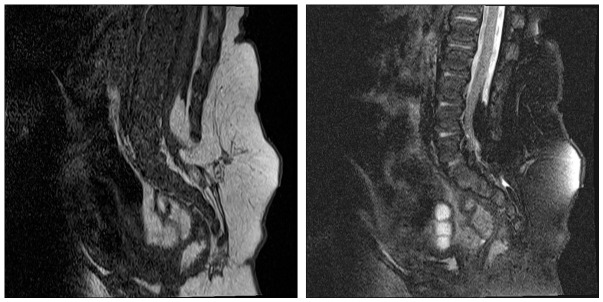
Pre-operative sagittal magnetic resonance imaging of the lumbosacral spine. Left: T1-weighted image, demonstrating the high-signal subcutaneous lipoma extending posteriorly from the spinal canal through a fascial defect; the lipoma is in continuity with intradural fat that attaches to a low-lying, tethered neural placode. Right: T2-weighted image of the same patient, demonstrating the conus medullaris position, the cord–sac relationship, and the cerebrospinal fluid-containing subarachnoid space.
